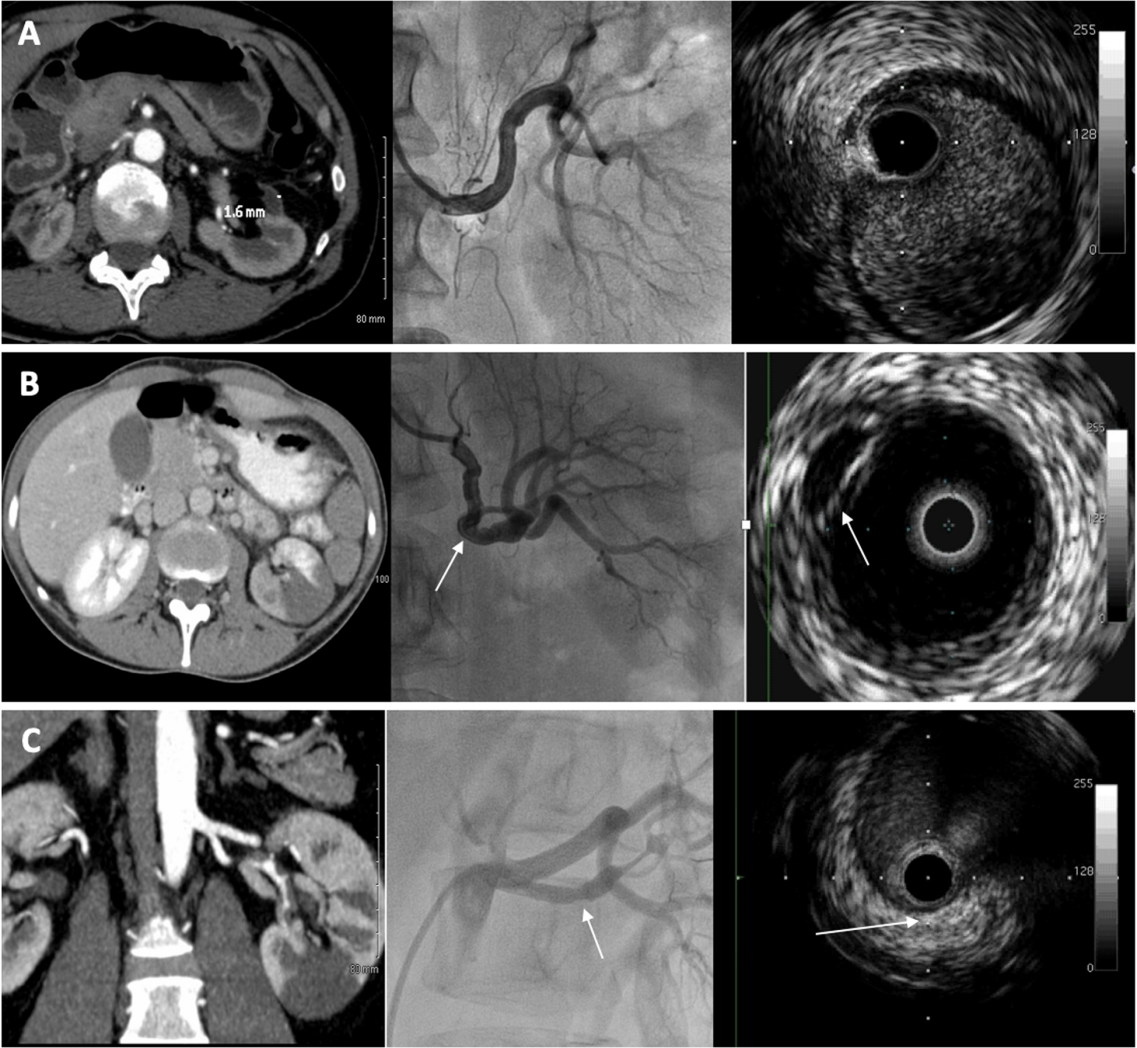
Examples of typical images in renal infarction patients. For each panel, the left image is a CT scan image (or reconstruction), the middle is the renal arteriography and the right image is an IVUS image. Panel a shows images from a patient with left renal infarction and no anomalies detected during the invasive exploration. Panel b shows images from a patient with left renal infarction and a spontaneous dissection visible on both renal angiography and IVUS (white arrow). Panel c shows images from a patient with left renal infarction and at least one calcified atherosclerotic plaque visible on both renal angiography and IVUS (white arrow)
